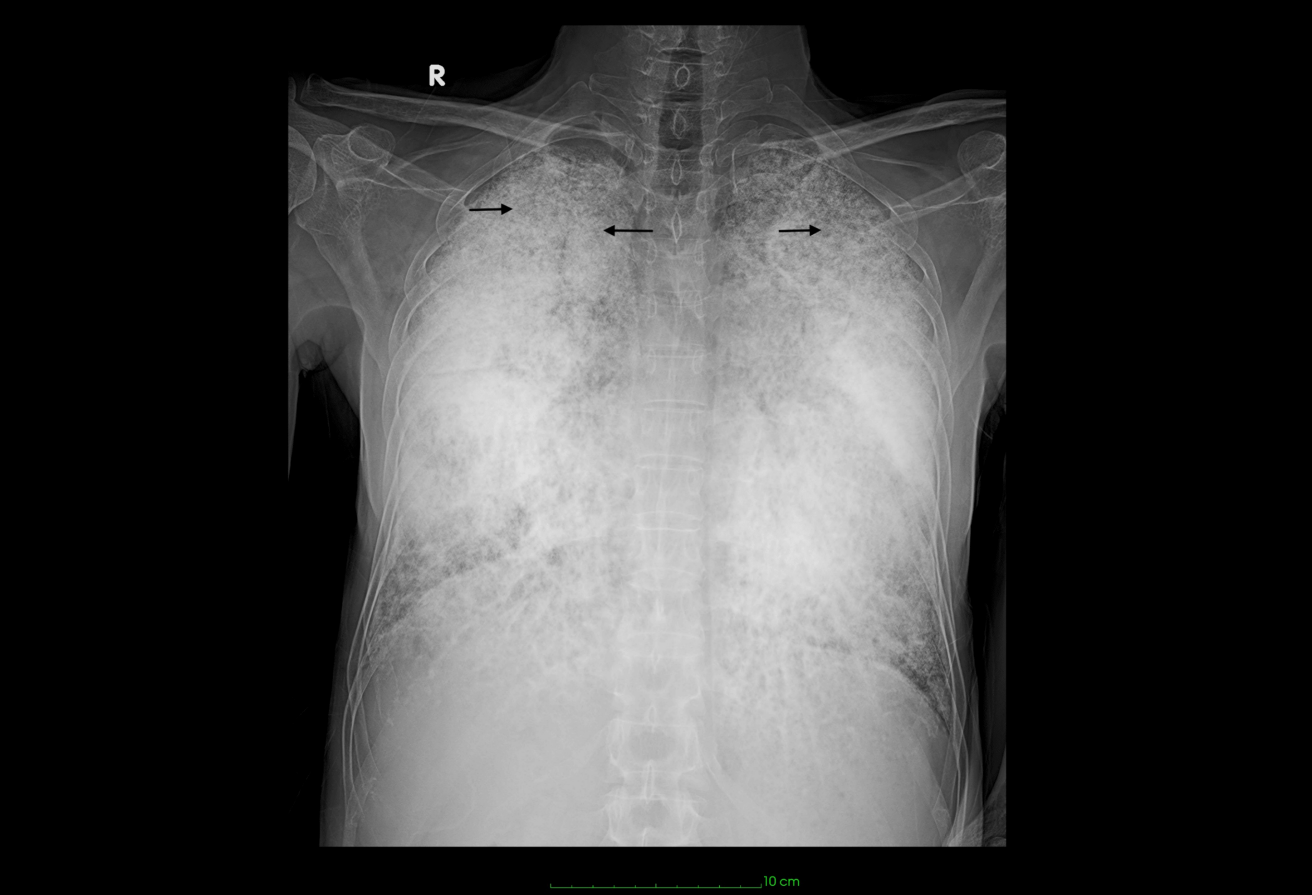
Chest X-ray showing extensive bilateral dense calcific opacities
